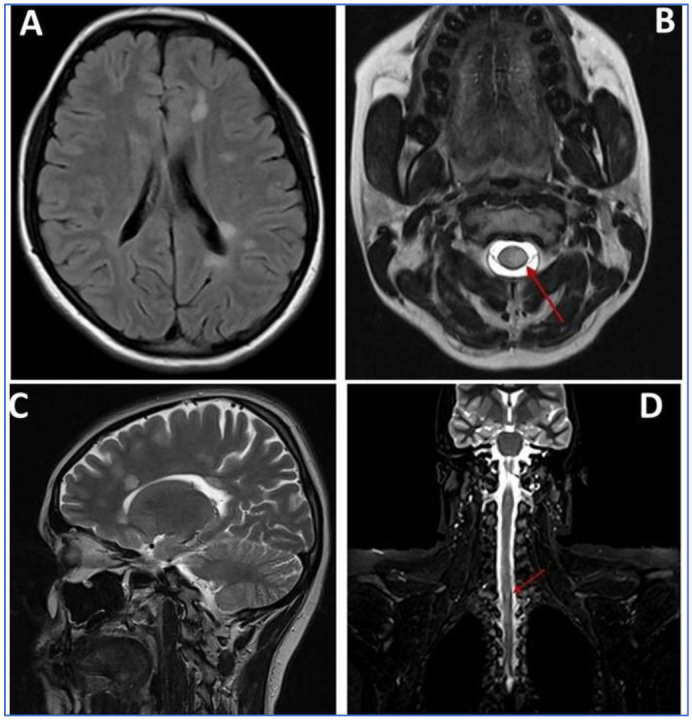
MRI scan images (A–D): (A) axial T2-weighted FLAIR MRI through the brain at the level of the ventricles showing multiple well-defined foci of increased signal intensity seen adjacent to left posterior aspect of periventricular white matter; (B) fat-suppressed axial T2-weighted MRI showing lesion around the spinal cord (Red Arrow); (C) sagittal T2-weighted MRI through the brain at the level of the ventricles showing multiple well-defined foci of increased signal intensity seen adjacent to the anterior aspect of periventricular white matter; (D) coronal T2-weighted MRI through the spinal cord showing well-defined foci of increased signal intensity (Red Arrow).
